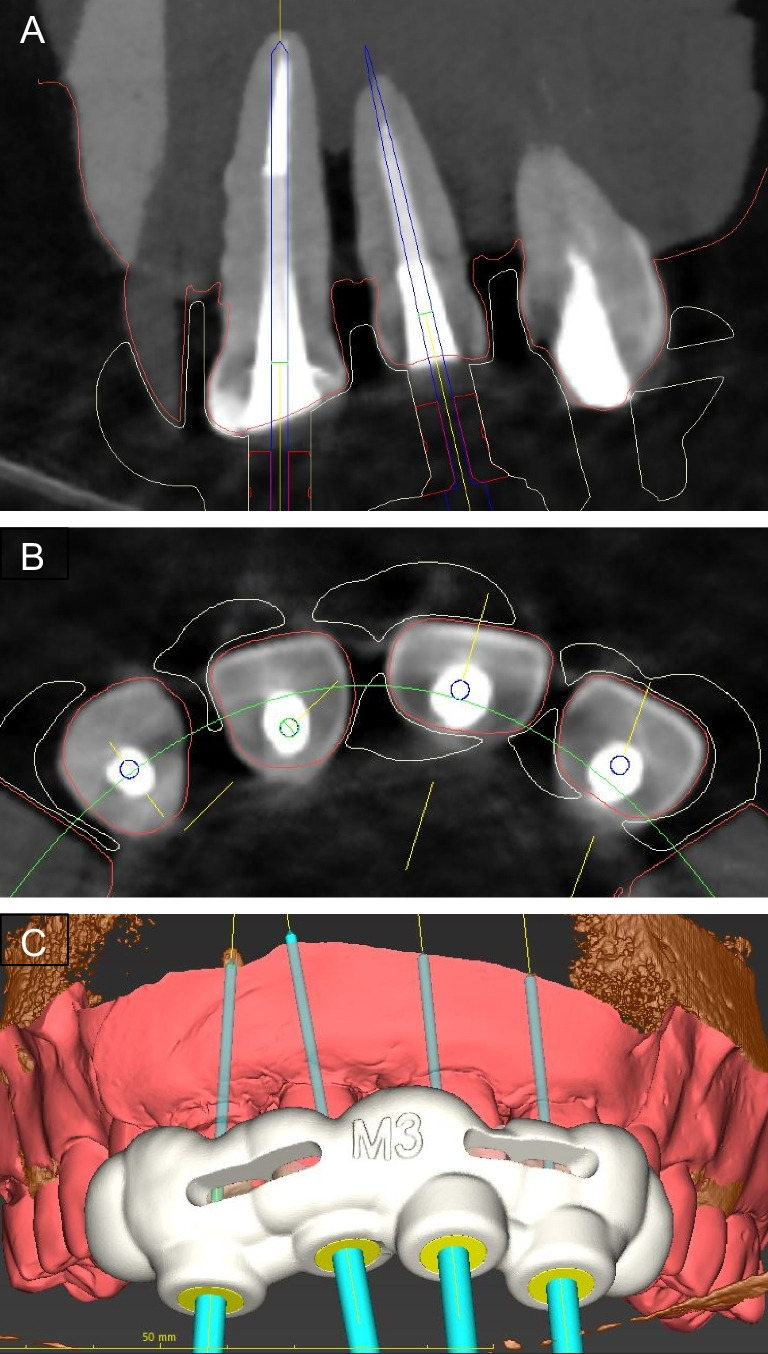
The guided endodontics technique with a virtually planned drill path targeting the root canal filling: coronal view (A), axial (B) view, and 3D rendered view of the matched surface scan and CBCT data with four planned access cavities and the designed template (C)
